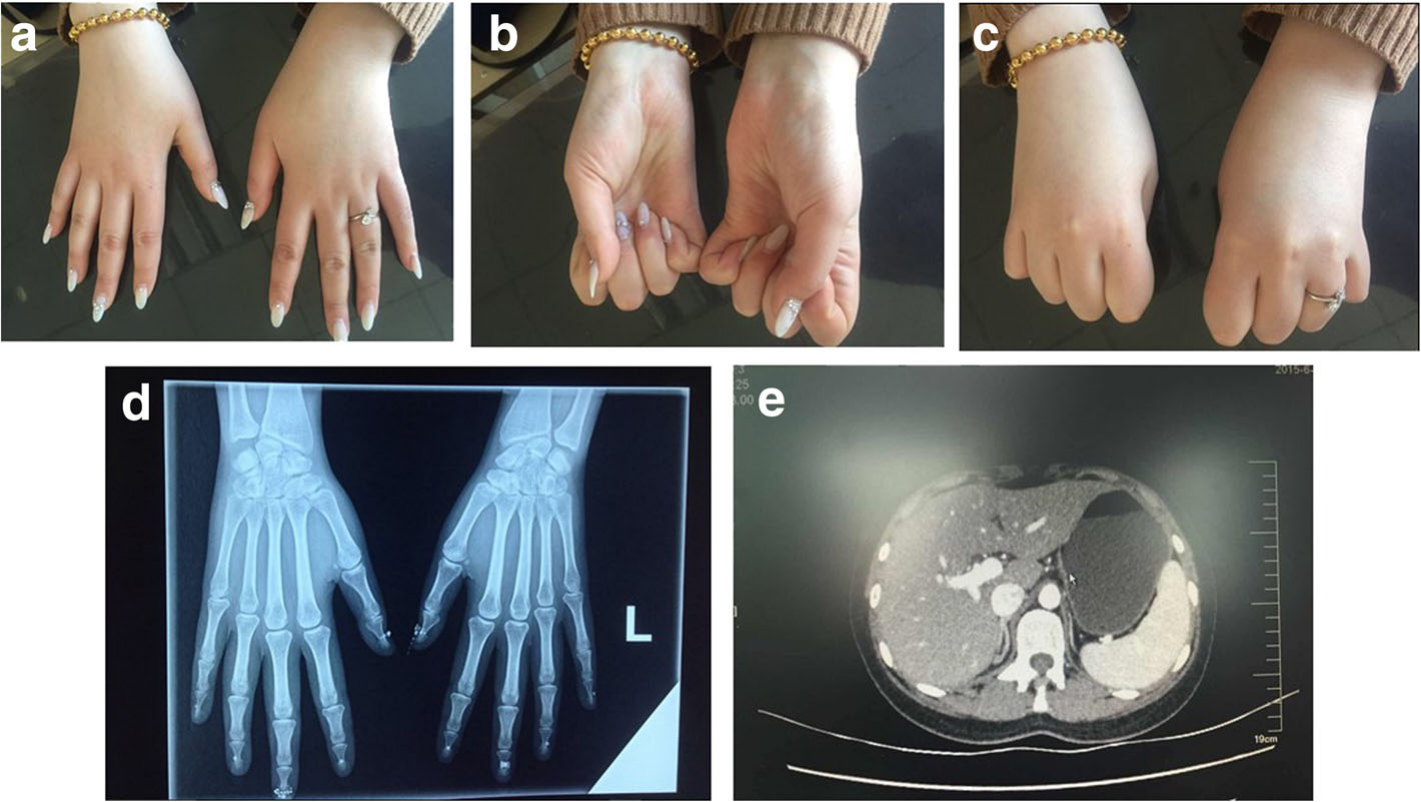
The appearance of the hands of the patient, hand DR and adrenal CT scans. a-c shows patient’s difficulty of bending the metacarpophalangeal joints, however her hand DR (d) suggested there were no bone abnormalities. The adrenal CT scan (e) showed there were no obvious hyperplasia changes
